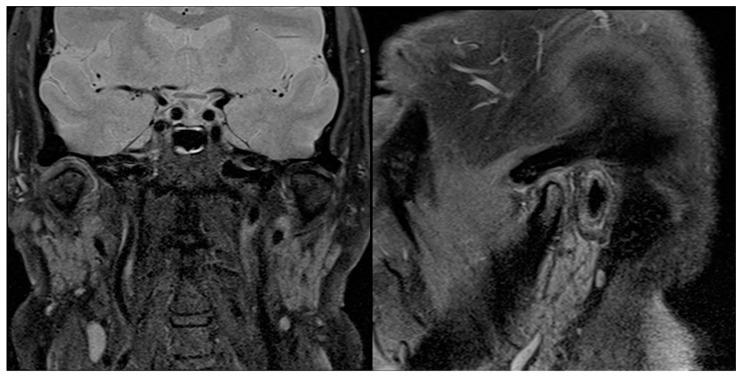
The 6-month postoperative sagittal and coronal MRI scans showing no signs of relapse on the right TMJ.
